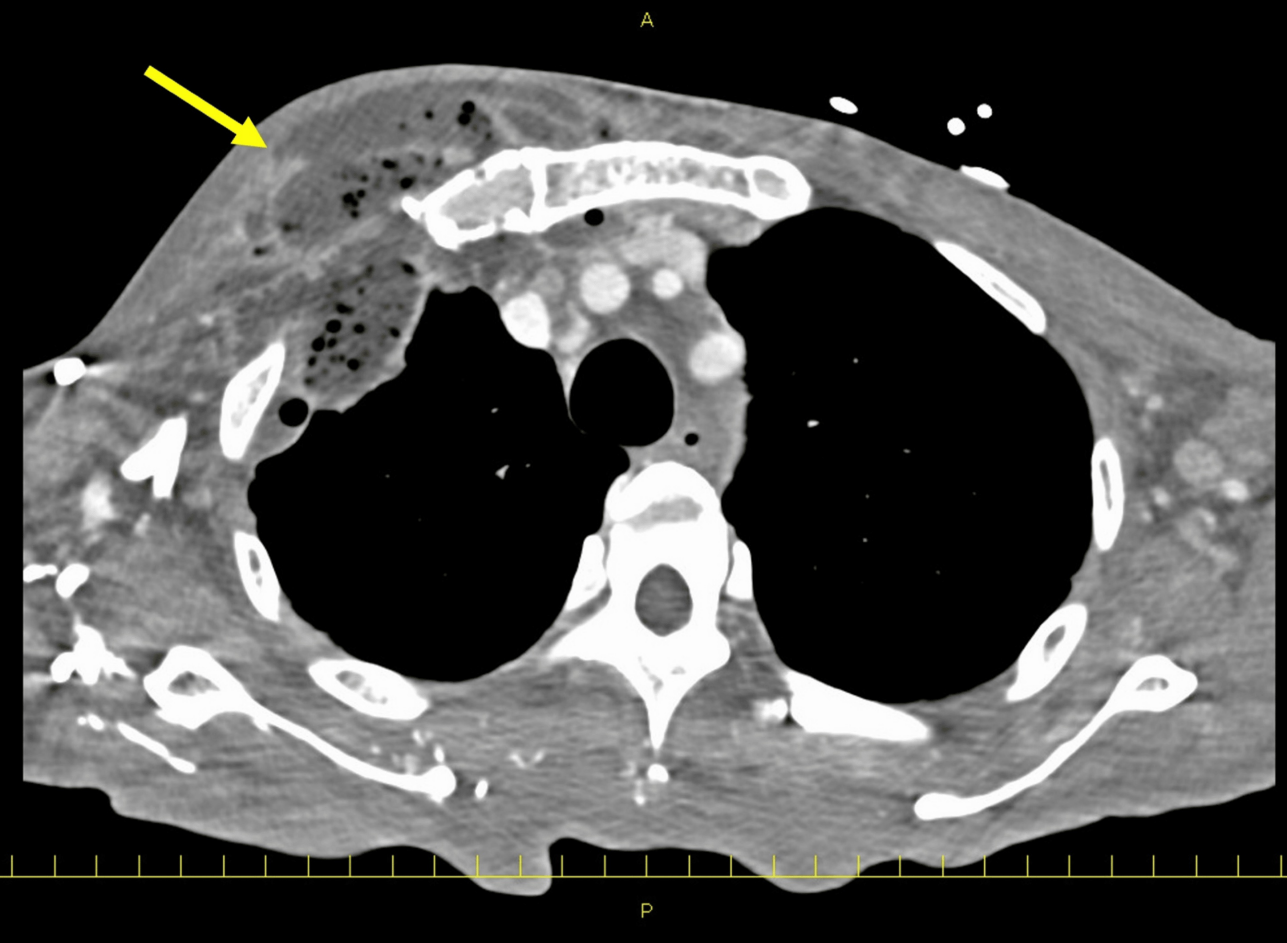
CT image at initial presentation An abscess (yellow arrow) was observed extending from the subcutaneous tissue of the right anterior chest to the thoracic cavity and the mediastinum behind the sternum. The first costal cartilage was dissolved and disconnected.
